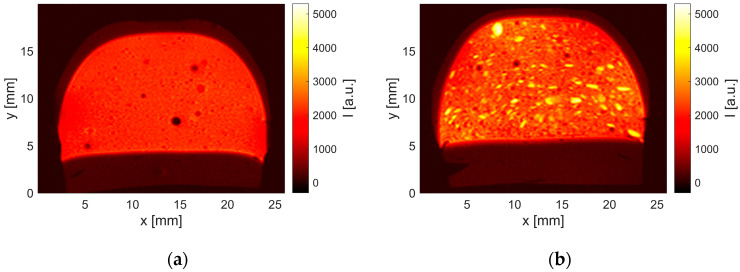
MR image of a fresh praline with a (a) fine ground (praline 1) and (b) coarse ground filling (praline 12), revealing typical transverse relaxation properties of the constituents. Nut pieces appear with signal intensities of 4000–5000 a.u., the filling is in the range of 2500 a.u., while the shell appears with signal intensities of 500–1000. The surrounding of the praline and small air bubbles in the filling have signal intensities on the noise level well below 120 a.u., when using the MRI parameters in Table 9.
